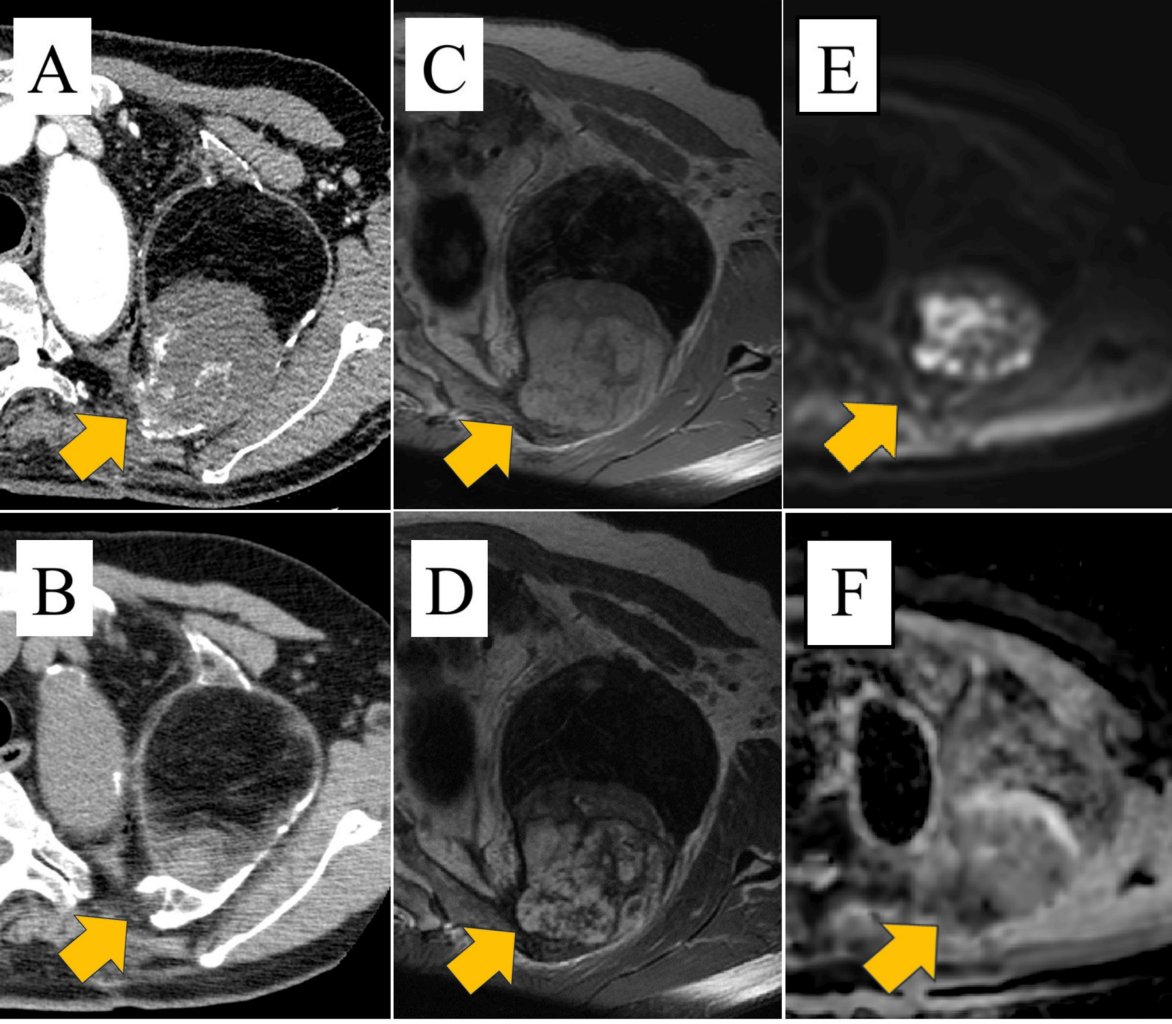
Chest CT and chest MR findings (A) Chest contrast-enhanced CT scan showing a plombage space with a 120 × 90 × 70 mm plombage space and a mass partly accompanied by a strong contrast effect in the plombage space (arrow). (B) A chest plain CT scan showed an 81 × 81 × 63 mm plombage space, and the size of the mass was 24 × 18 × 18 mm three years ago (arrow). (C) T1-weighted MR image showing a low-to-slightly high-intensity mass in the plombage space (arrow). (D) In the T2-weighted MR image, the mass had a low to high intensity and a mosaic-like appearance (arrow). (E) Diffusion-weighted imaging showed a mosaic appearance ranging from high to low signal intensity (arrow). (F) The apparent diffusion coefficient (ADC) map also showed a mosaic appearance ranging from high to low signal intensity (arrow).
